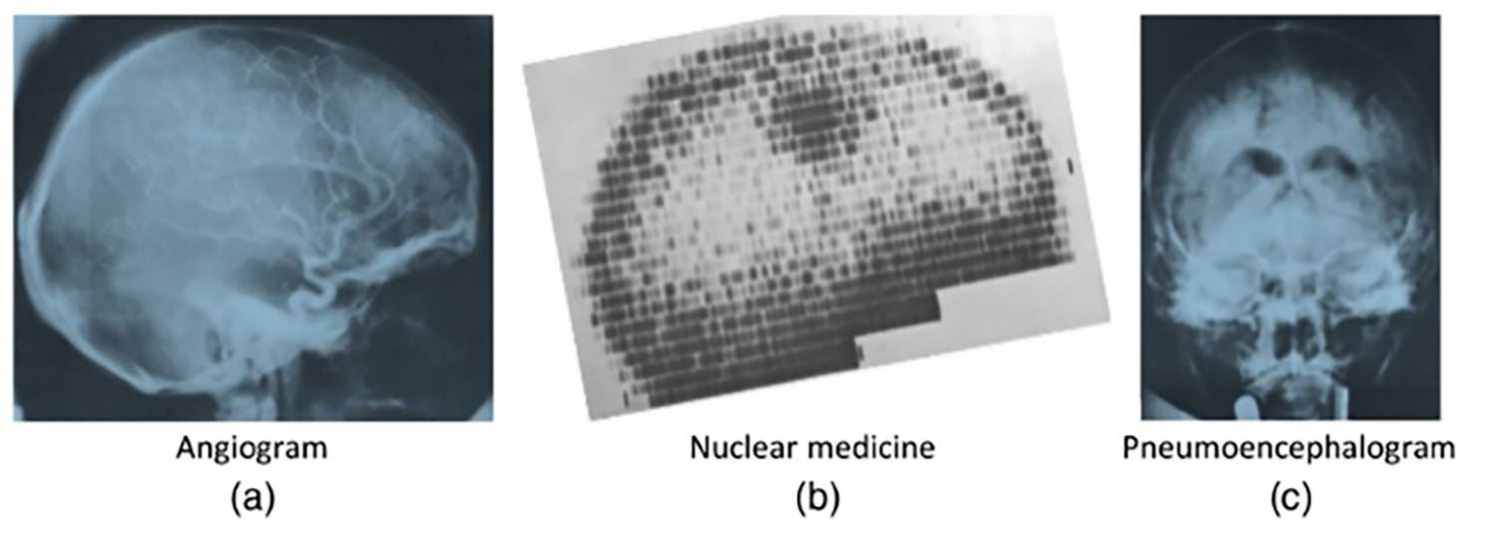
CT replaced numerous invasive procedures in neurology such as angiogram (a), nuclear medicine scans (b) and pneumoencephalograms (c). Photographs are included in the EMI, Ltd., “New EMI machine for diagnosing brain disease,” First EMI Brochure (April 1972)
